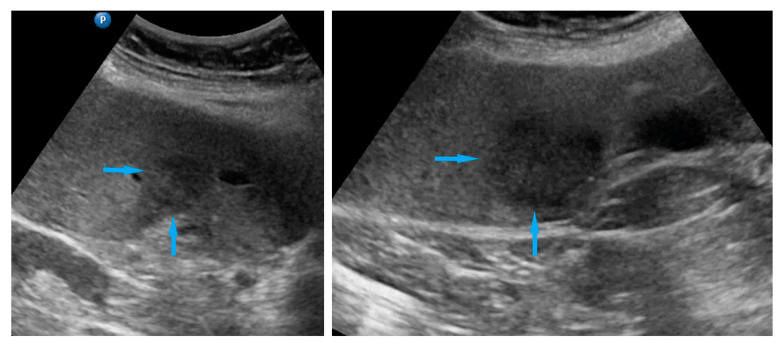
Ultrasound images of hepatic parenchymal lesions. Blue arrows identify tumors.
